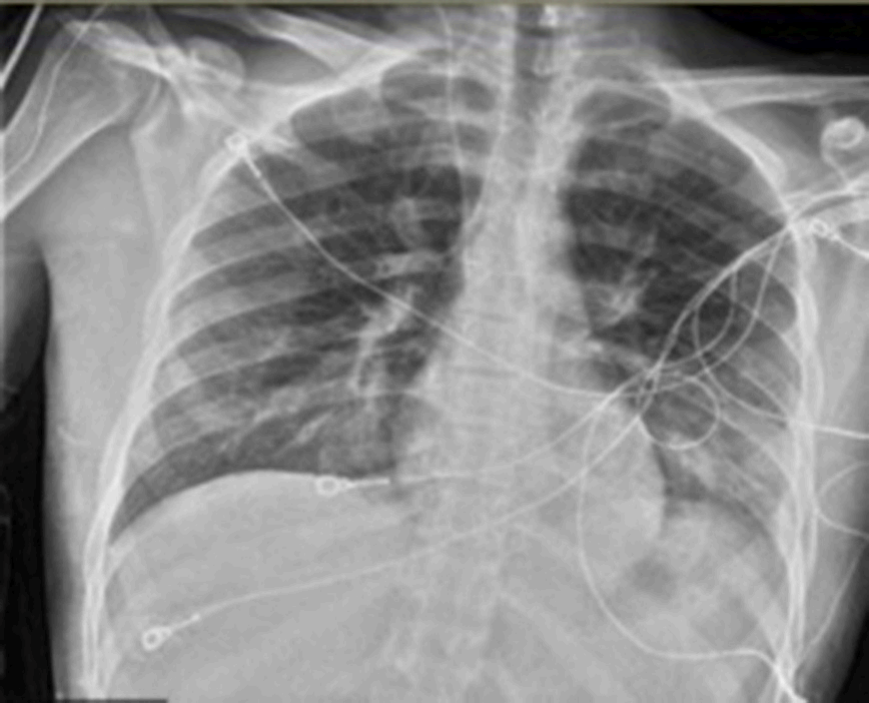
Chest radiograph immediately following syncopal episode and hypoxia.
